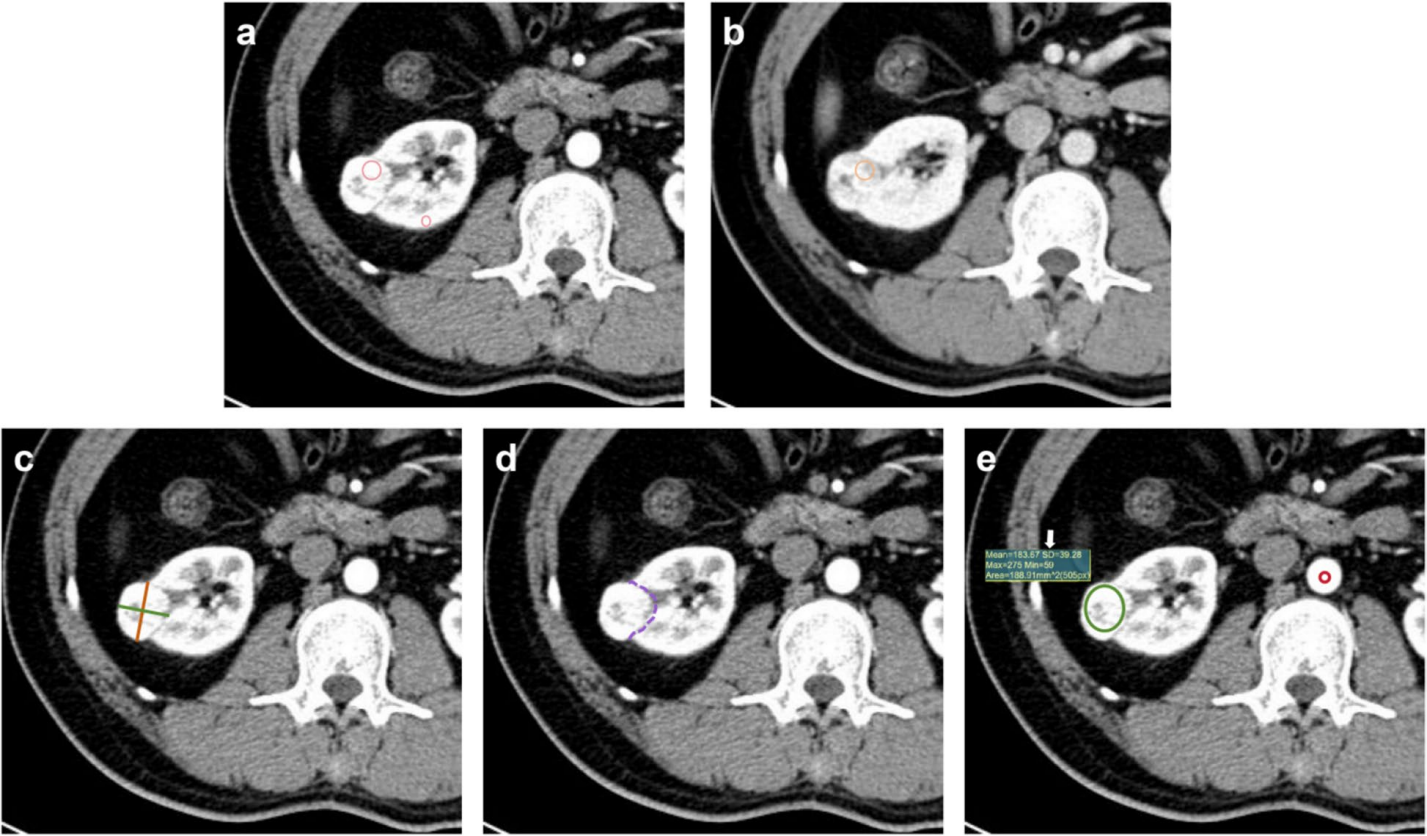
a Axial CT images of corticomedullary phase (CMP), placed a region of interest (ROI) in the area where the mass showed the most enhancement (large circle), and another standard ROI in the cortex of the ipsilateral renal (small circle), then recorded the mass-to-cortex corticomedullary attenuation ratio; b Axial CT images of nephrographic phase (NP), copied the ROI of the mass on the CMP image to the NP image at the same slice, to attenuation value in NP and determine standardized nephrographic reduction rate; c Axial CT images of CMP, measured the maximum major axis and minor axis (mutually perpendicular) of the mass to determine the Major axis / Minor axis; d Axial CT images of CMP, recorded tumor-renal interface that the maximum curved surface length of the mass in contact with the renal parenchyma (dotted line); e Axial CT images of CMP, place a ROI (green circle) on largest slice of the mass and its adjacent superior and inferior slices, and placed corresponding ROIs (red circle) on the aorta determine to the standard deviation of attenuation value (arrow) and the standardized heterogeneity ratio
